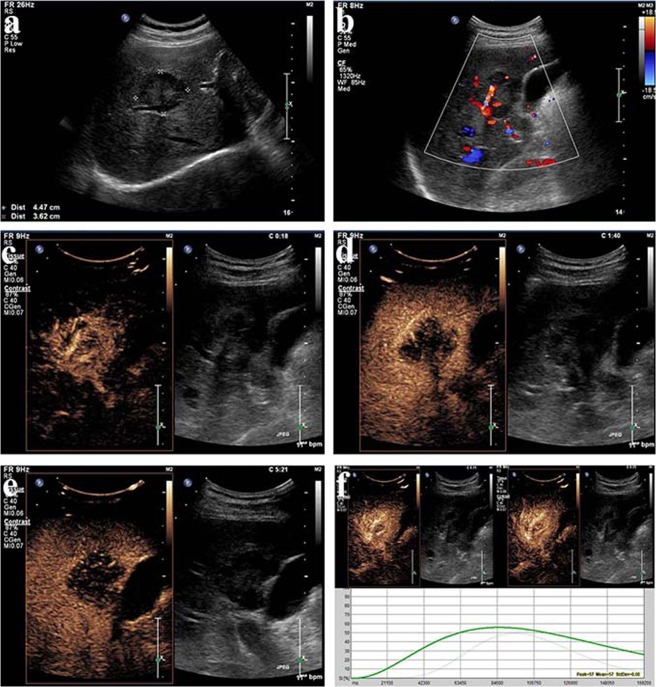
Intrahepatic cholangiocarcinoma (ICC) in a 54–year-old male. (see Supplementary Video S2). (a) Conventional ultrasound shows a hypoechoic lesion with a size of 4.5 cm × 3.6 cm near the hepatic hilar region (cursors); (b) Color Doppler sonography indicates a relatively abundant blood supply; (c) During the arterial phase (18 s), the lesion exhibits heterogeneous hyperenhancement; (d) During the portal venous phase (100 s), the lesion exhibits hypoenhancement of its interior with hyperenhancement in the peripheral region, resulting in the formation of a hyperenhanced rim; (e) During the late phase (321 s), the lesion exhibits hypoenhancement; (f) The parametric curves reveal perfusion of the region of interest in the interior (light green, Peak = 50.0, Sharpness = 0.105 1/s) and periphery (dark green, Peak = 56.8, Sharpness = 0.030 1/s) of the lesion from 10 s to 180 s.
